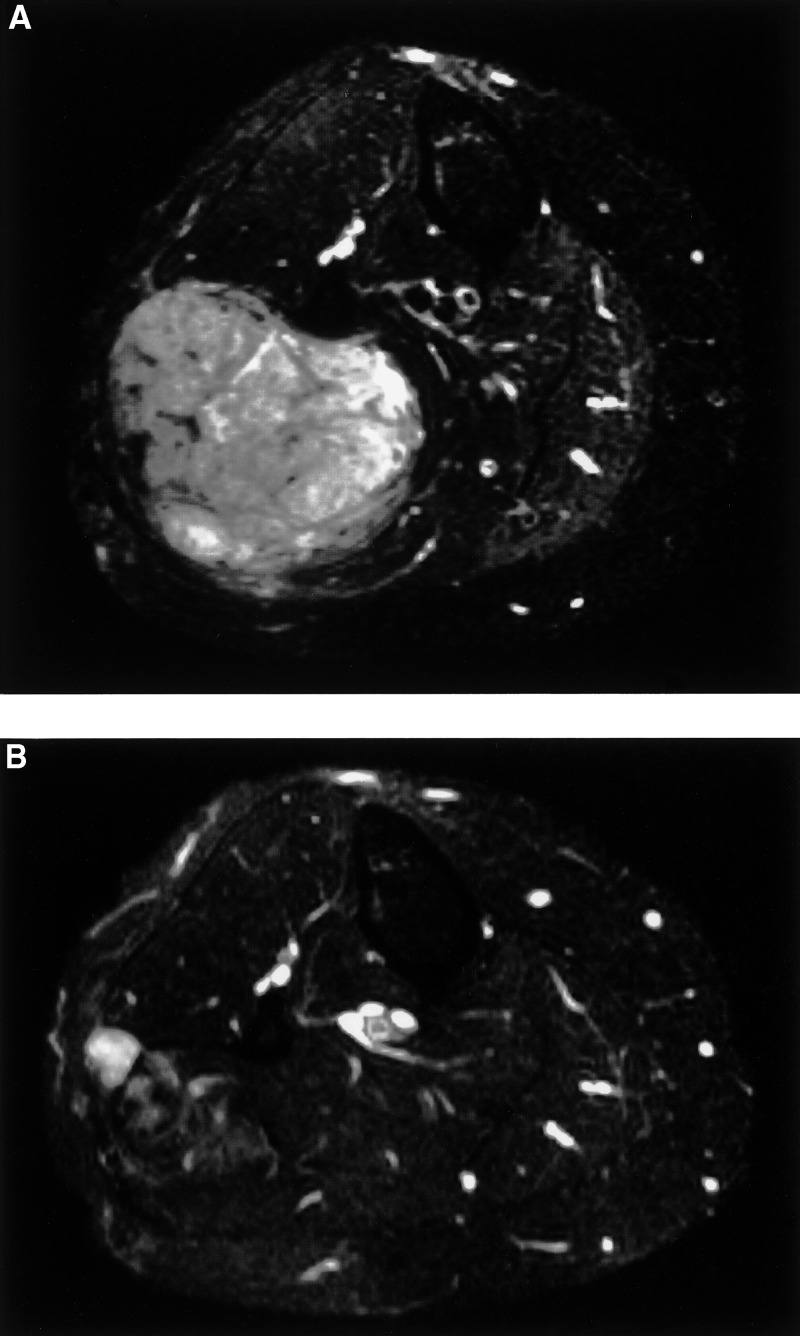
Fat-suppressed T2-weighted axial MR image after three courses of chemotherapy (A). A relatively well-defined mass of heterogeneous high signal intensity is shown in the right calf. Compared with the prechemotherapy MR examination (not shown), a small increase in tumour volume was noticed. After treatment with IFN alpha-2b, there is an obvious decrease of the tumour in the right calf with an overall decrease in signal intensity and a focal remnant area of high signal intensity on the lateral side (B).
